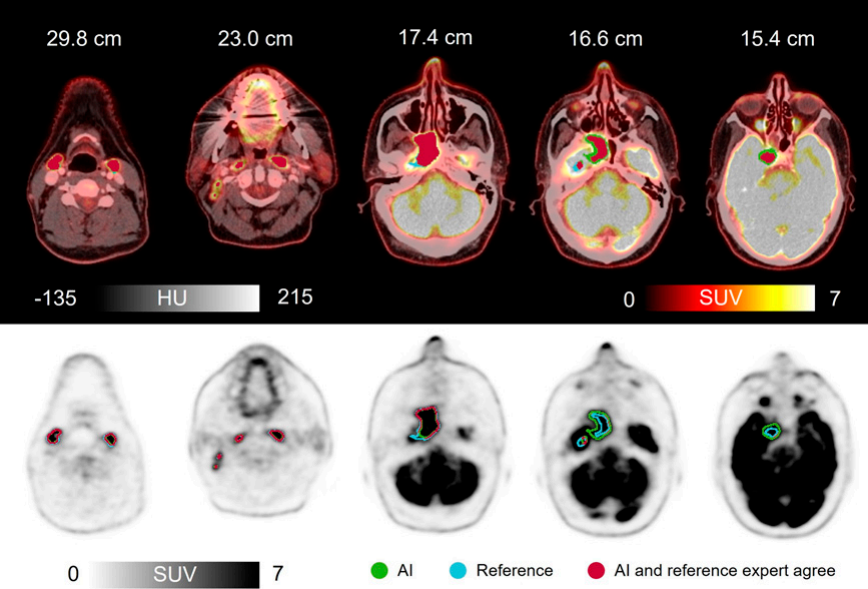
Clinical scan delineated by expert (reference) and by AI, along with AI-to-expert agreement (DSC, 0.92). Shown are axial images of 50-y-old man with HNC of rhinopharynx. ^18^F-FDG PET/CT with intravenous contrast agent showed greatly increased activity corresponding to large tumor process in right rhinopharynx, crossing midline and growing frontally into cavum nasi on right, intracranially on right, medially in fossa media, and along dura laterally. In addition, multiple lymph nodes in neck had greatly increased activity bilaterally. AI correctly avoided including physiologically active areas such as saliva, metal artifact–induced activity, nose tip, brain, and optic nerve. HU = Hounsfield units.
